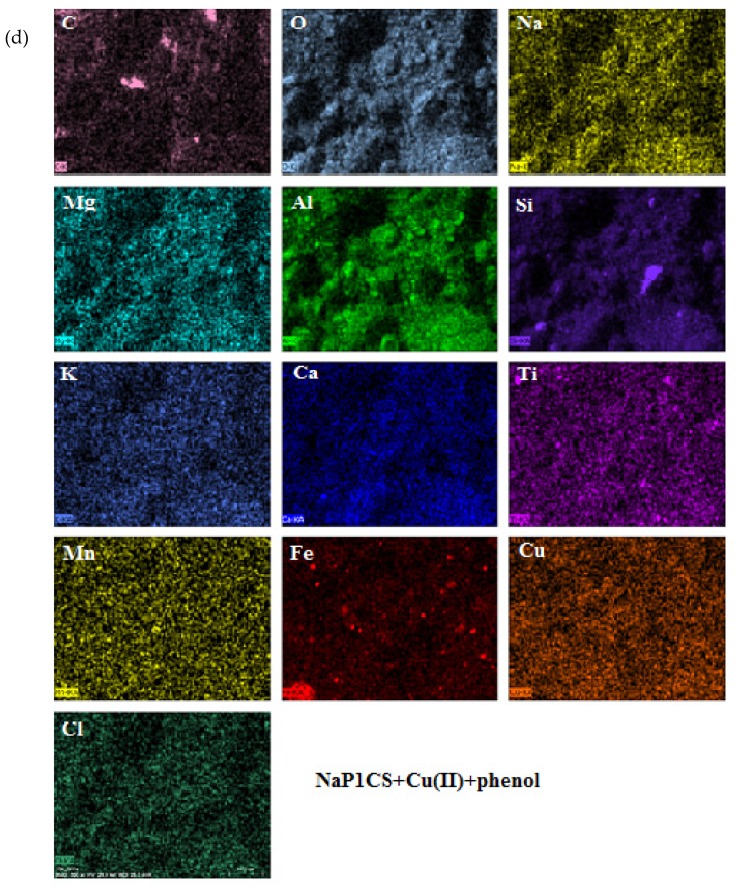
SEM–EDS analysis of (a) NaP1 and (b) NaP1CS after sorption of Cu(II) and phenol with the corresponding X-ray maps of C, O, Na, Mg, Al, Si, K, Fe, Ti, Mn, Cu, Ca, P, and S on (c) NaP1, and (d) NaP1CS (magnification: 1000×).
